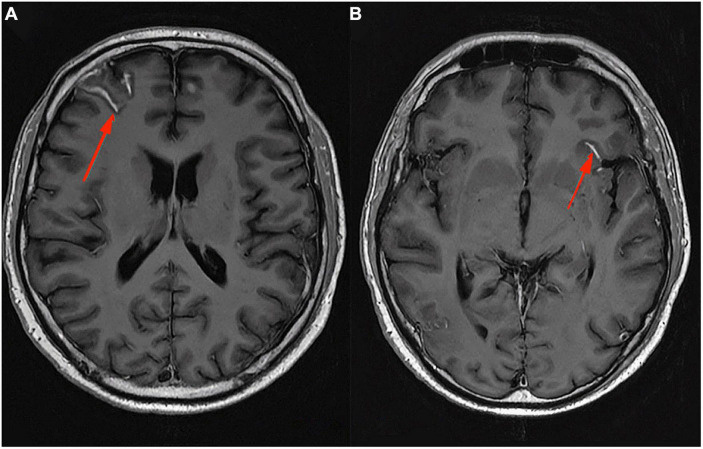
MRI T1-weighted sequence with contrast, axial section. Leptomeningeal contrast enhancement in a patient with SPMS (A,B). Material from the resources of the Medical Radiology Department of the Military Medical Institute–National Research Institute.
